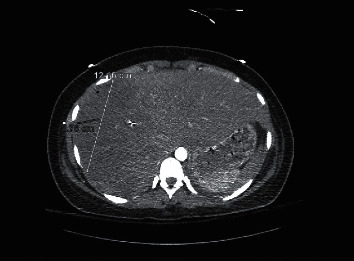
CTA abdomen on postoperative day 4 showing improvement in size and no active arterial extravasation.
